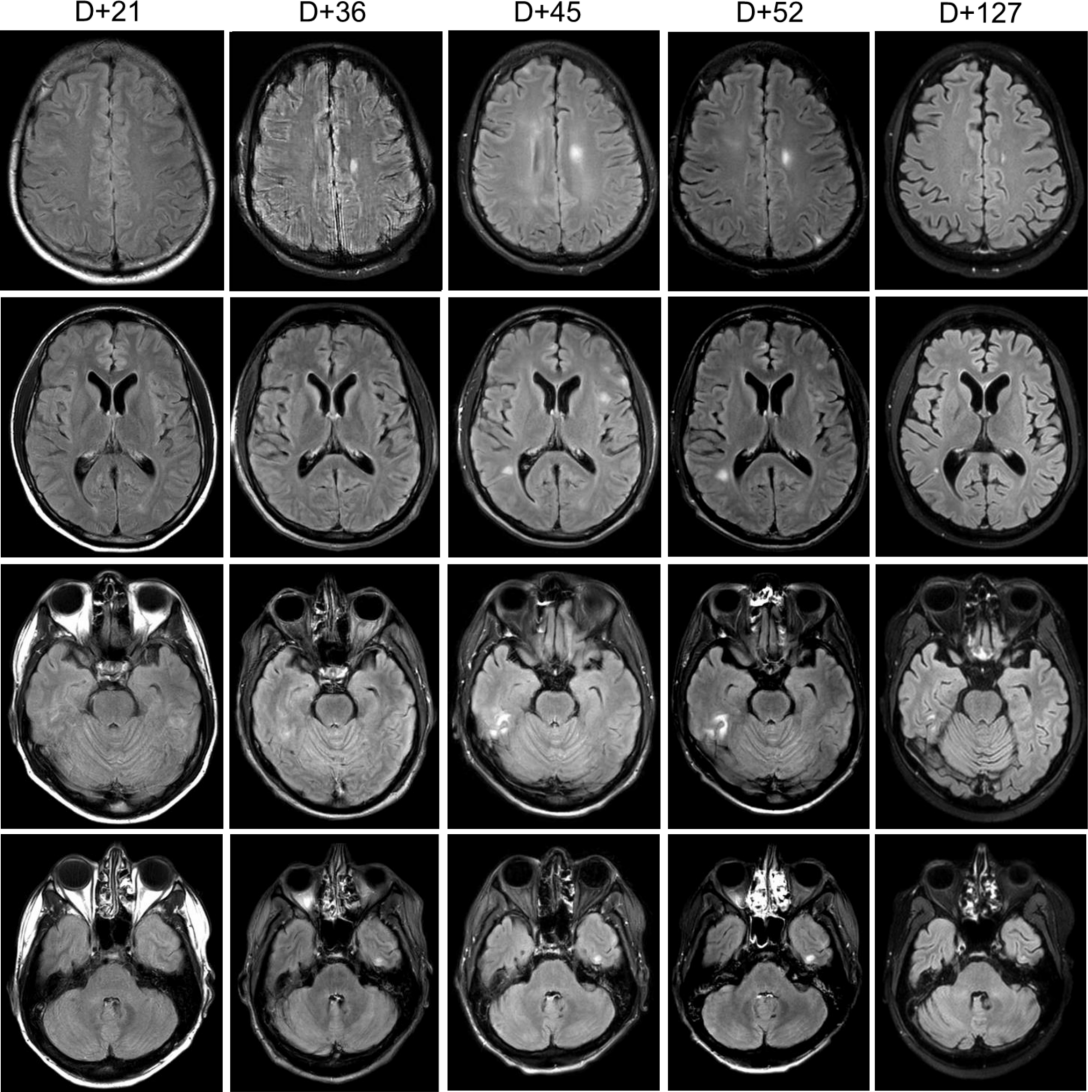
Brain MRI. Serial sections of T2-weighted fluid-attenuated inversion recovery (FLAIR) MRI brain sequences noting evolution areas of cortical enhancement. “D+” denotes day post-HSCT.
